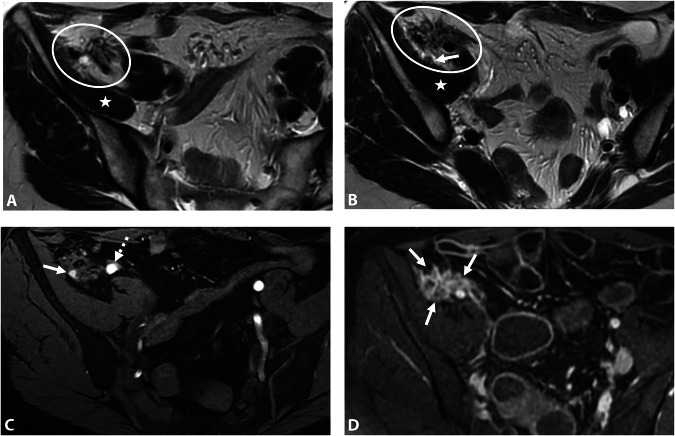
Femoral nerve endometriosis in a 34-year-old woman. A, B Axial 3D reconstruction T2W MR images show a fibrotic and retractile infiltration (circles) in contact with the right iliacus muscle (stars), on the course of the right femoral nerve (arrow). C Axial 3D reconstruction fat-suppressed T1W MR image reveals some T1-hyperintense endometriotic hemorrhagic microcysts (arrow), not to be confused with the external iliac artery (dashed arrow). D Axial T1W post-contrast MR image shows persistent enhancement of the lesion (arrows)
